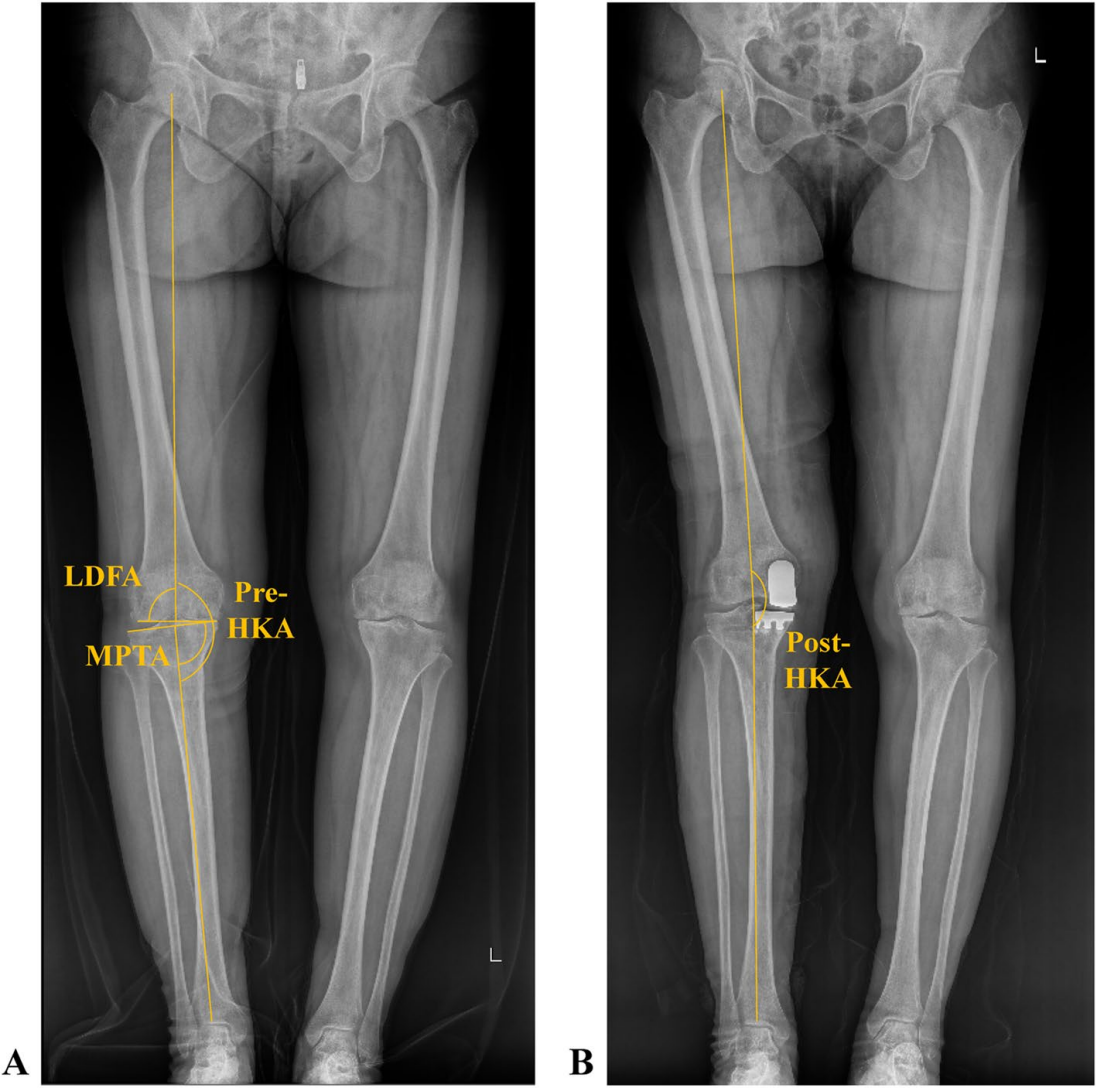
Measurement of different alignment parameters on the weight-bearing long-leg radiograph. Pre-HKA, preoperative hip-knee-ankle angle; post-HKA, postop hip-knee-ankle angle; LDFA, lateral distal femoral angle; MPTA, medial proximal tibial angle
